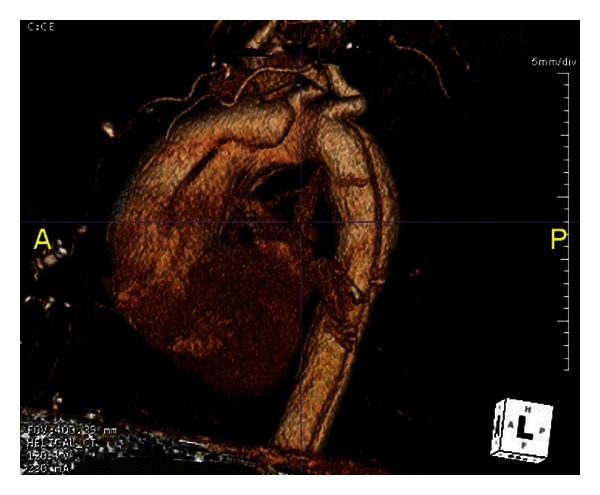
CTA reconstruction demonstrating acute dissection in ascending and descending aorta.
